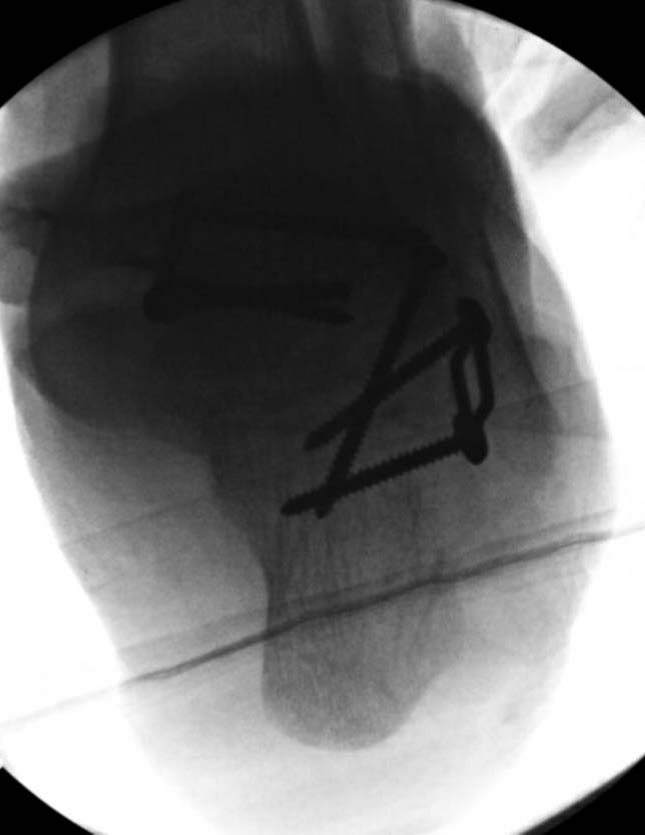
Hindfoot radiograph of the foot intraoperatively after the hexapod frame was removed and arthrodesis was completed.
